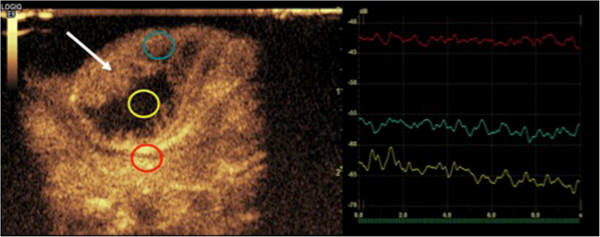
Contrast-enhanced ultrasound (CEUS) with intravascular SF_
